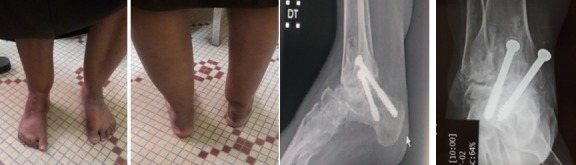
Aspect clinique + radiographie à 3 ans PO
